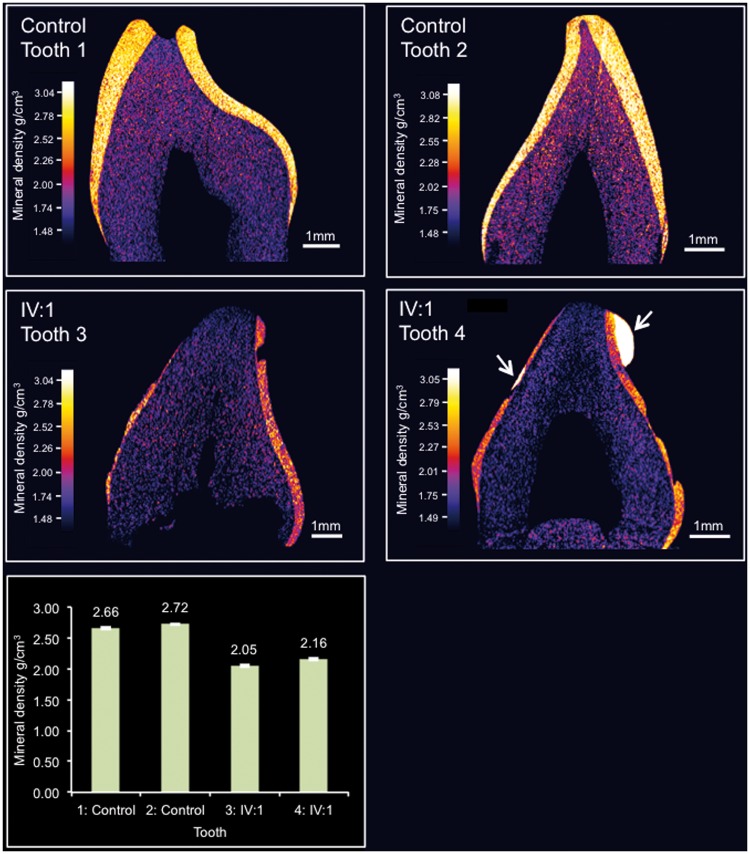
High resolution X-ray CT analysis of exfoliated teeth from control individual and individual IV:1. Typical CT sections through the teeth are presented using false colour calibrated with respect to mineral density to generate mineral density maps. Mean enamel mineral density for each tooth is also shown graphically, error bars represent the twice the standard error of the mean. The teeth from the control individuals (Teeth 1 and 2) exhibit an enamel layer apparently normal in structure and density. Teeth 3 and 4 from IV:1 exhibit an obvious enamel covering though it is thinner, chipped, absent in places and is reduced in mineral density compared with teeth 1 and 2. Note that tooth 4 has undergone restoration (indicated by arrows). Videos of 3D rendered CT data showing surface detail and internal structure of teeth 1-4 are available as Supplementary Material.
